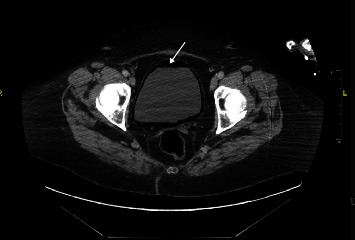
CT abdomen and pelvis showing emphysematous cystitis.
